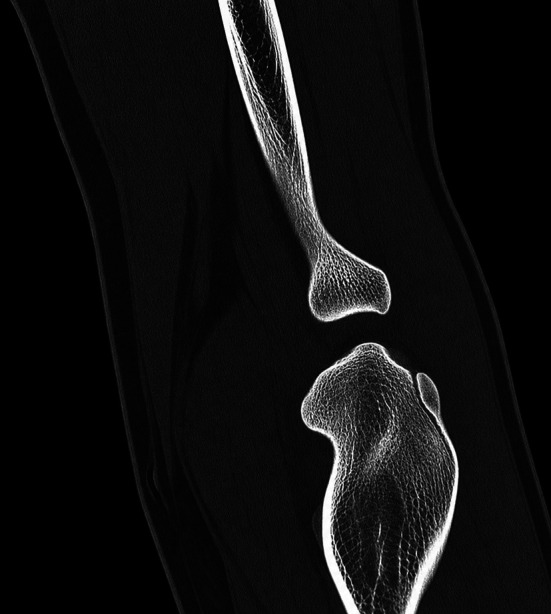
Three-month postoperative computed tomography demonstrating osseous consolidation of the capitellar fragment with restoration of articular congruity and maintained reduction.
